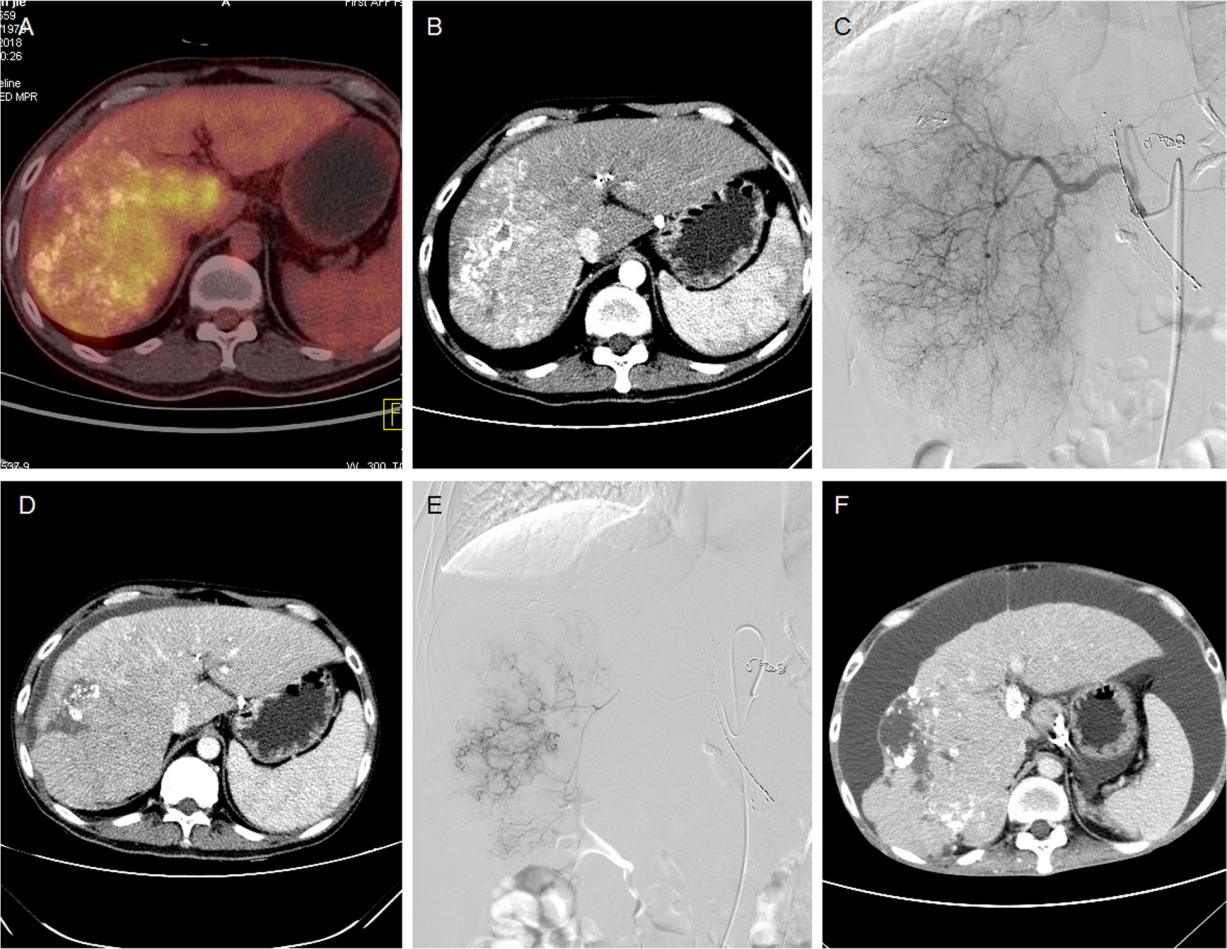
A 41-year male treated by DEB-TACE for advanced primary HCC. A-B CT revealed a large tumor of 133.2 mm in right liver one month after conventional TACE. C The tumor artery was superselectively incubated and embolized by 300-500 μm raltitrexed-loaded beads. D CT revealed a shrunk tumor 1.3 months after first DEB-TACE. E A second DEB-TACE was performed with 300-500 μm raltitrexed-loaded beads. F The tumor was found to shrink after 5.1 months' follow-up, but died of disease progression 7.5 months later
